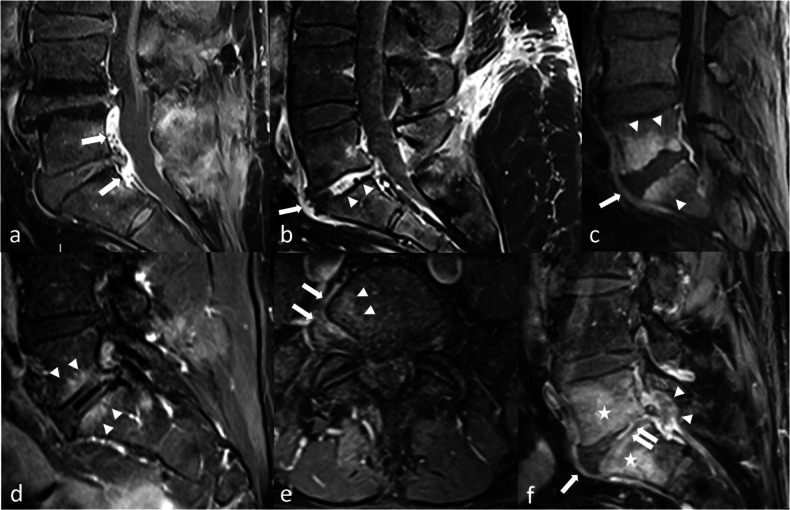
a Normal enhancement of the anterior epidural space (white arrows) after disc surgery on post-contrast fat-suppressed sagittal T1-weighted MRI images. b In this other patient with mild postoperative back pain after disc herniation removal, post-contrast fat-suppressed sagittal T1-weighted MRI images showed an expected disk enhancement (arrowheads), but also an atypical pre-vertebral enhancement (arrow). After negative biology and close follow-up, infection was ruled out and the patient recovered. c Classic aspect of haematogenic L5–S1 spondylodiscitis with oedema and extensive enhancement of both vertebral end-plates (arrowheads) and prevertebral tissues (arrow) on MRI fat-suppressed sagittal T1-weighted images (c). d–f Another patient benefited from L5-S1 laminectomy and intra-canalar facet joint cyst removal. MRI was performed because of mild postoperative lumbar pain associated with mild inflammatory syndrome. On sagittal (d) and axial (e) post-contrast fat-suppressed T1-weighted images, lateralised peri-discal oedema (arrowheads) extending in the adjacent soft tissues (arrows) was neglected, although this was atypical as these structures were not supposed to be concerned by surgery. The patient got worse and another MRI revealed features of spondylodiscitis with extensive peri-discal oedema (asterisks), and prevertebral (arrow), disk (double arrows), and foramino-epidural (arrowheads) enhancement: post-contrast fat-suppressed T1-weighted images (f)
